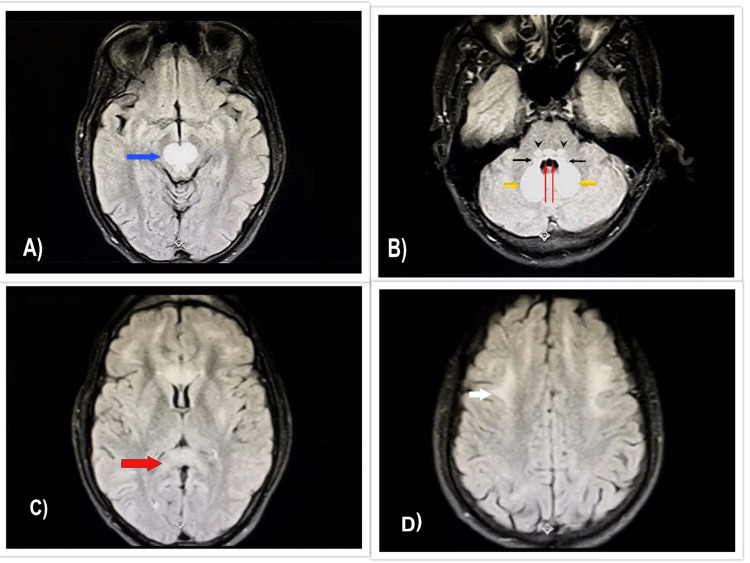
0.4 Tesla MRI and axial FLAIR images demonstrate bilaterally symmetrical hyperintense signals. (A) Midbrain involving red nuclei and tegmentum (blue arrow). (B) Cerebellar dentate nuclei (thick yellow arrows); dorsal pons showing vestibular (thin black arrows) and abducens nuclei (thin red arrows) involvement, while in caudal pons, superior olivary nuclei lesion is seen (arrowheads). (C) Corpus callosum (splenium) (thick red arrow). (D) Frontal lobe subcortical white matter (white arrow). MRI: magnetic resonance imaging; FLAIR: fluid-attenuated inversion recovery
